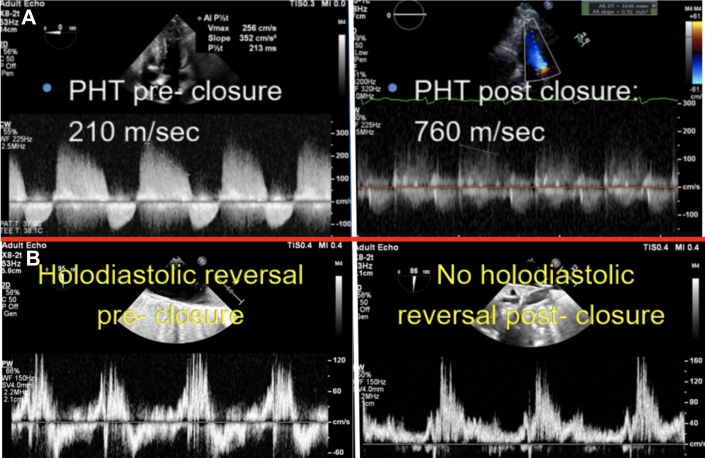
Echocardiographic Markers of Paravalvular Leak Improvement (A) Echocardiographic markers of aortic insufficiency, including pressure half time, improve from preclosure (210 ms) to postclosure (760 ms). (B) Holodiastolic reversal in the aorta is present before closure and normalizes after closure.
